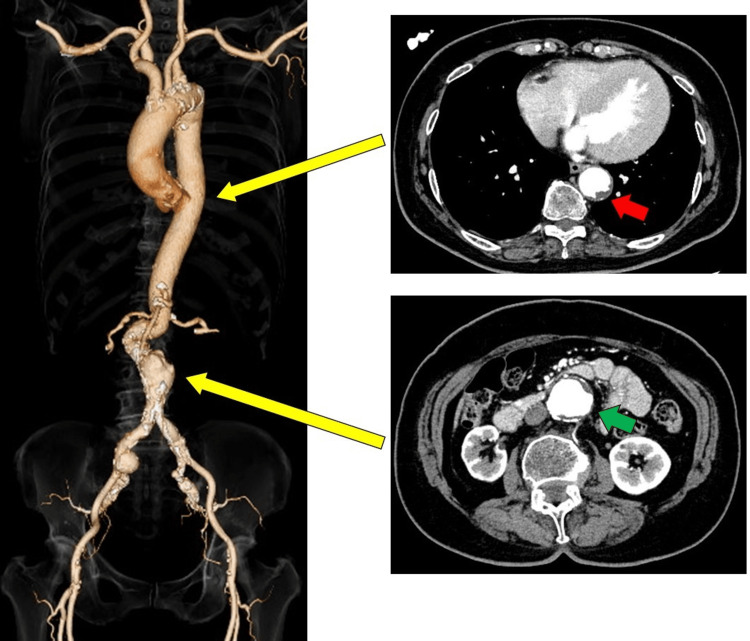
Computed tomography of the aorta of the present case The figure on the left is a 3D reconstructed image of the patient's aorta. The carotid artery had minimal calcification and enough diameter. The abdominal aorta with severe tortuosity and aneurysms were observed. The two figures on the right are axial cross-sectional images, showing representative findings that determined that transfemoral access was unsuitable. Yellow arrows indicated where each axial section corresponds to in the 3D reconstructed image. The red arrow indicated plaques in the descending aorta. The green arrow indicated abdominal aortic aneurysm and atherosclerotic plaque.
